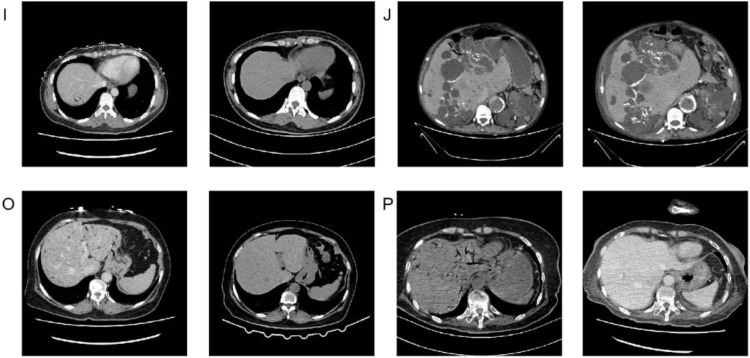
Case vignettes demonstrating temporal evolution of HPVG under conservative treatment. (I) HPVG related to gastroduodenal inflammation, complete resolution at day 6; (J) HPVG in a patient on chronic hemodialysis with ascites, complete resolution at day 3 after drainage and antibiotics; (O) Extensive HPVG resolving by day 10 on ultrasound; (P) HPVG with suspected ischemic colitis, complete CT resolution at day 84 after comprehensive medical therapy.
